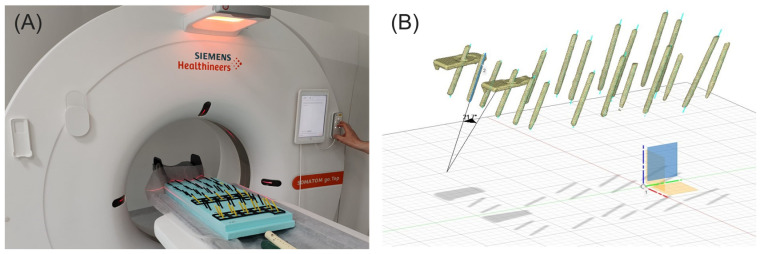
(A) CT scanning of the angle estimation experimental setup. (B) The obtained 3D model of the rods inserted into the holes to enable angle measurements.
